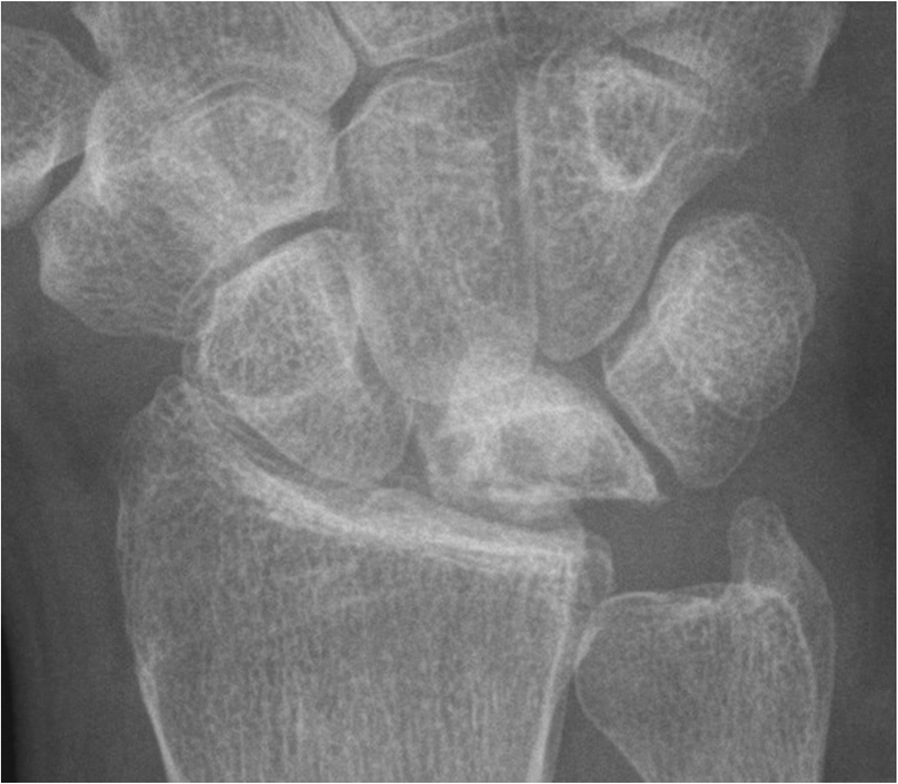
Pre-operative x-rays of the right wrist of of a 45-year-old male patient who had undergone STT arthrodesis
